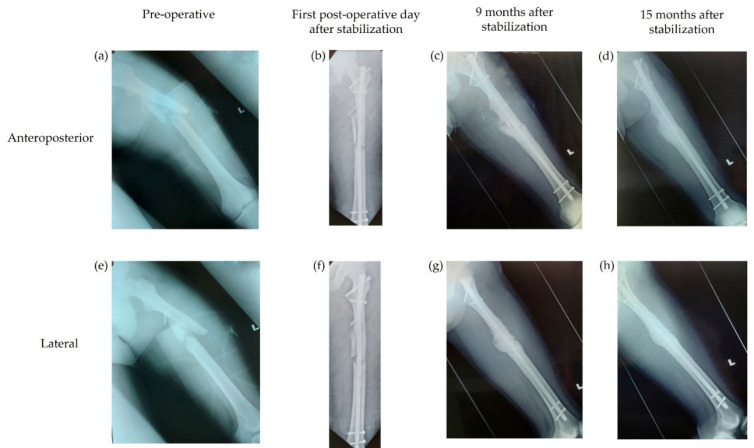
Example of delayed bony union, as assessed by the independent experts. Radiographs from an 18-year-old male patient with a Gustilo type IIIa open femoral fracture. (a–d) Anteroposterior radiographs. (e–h) Lateral radiographs. (a,e) Radiographs taken pre-operatively; (b,f) radiographs taken on the first post-operative day after stabilization of the fracture; (c,g) radiographs taken 9 months after stabilization; and (d,h) radiographs taken 15 months after stabilization that demonstrate bony union.
